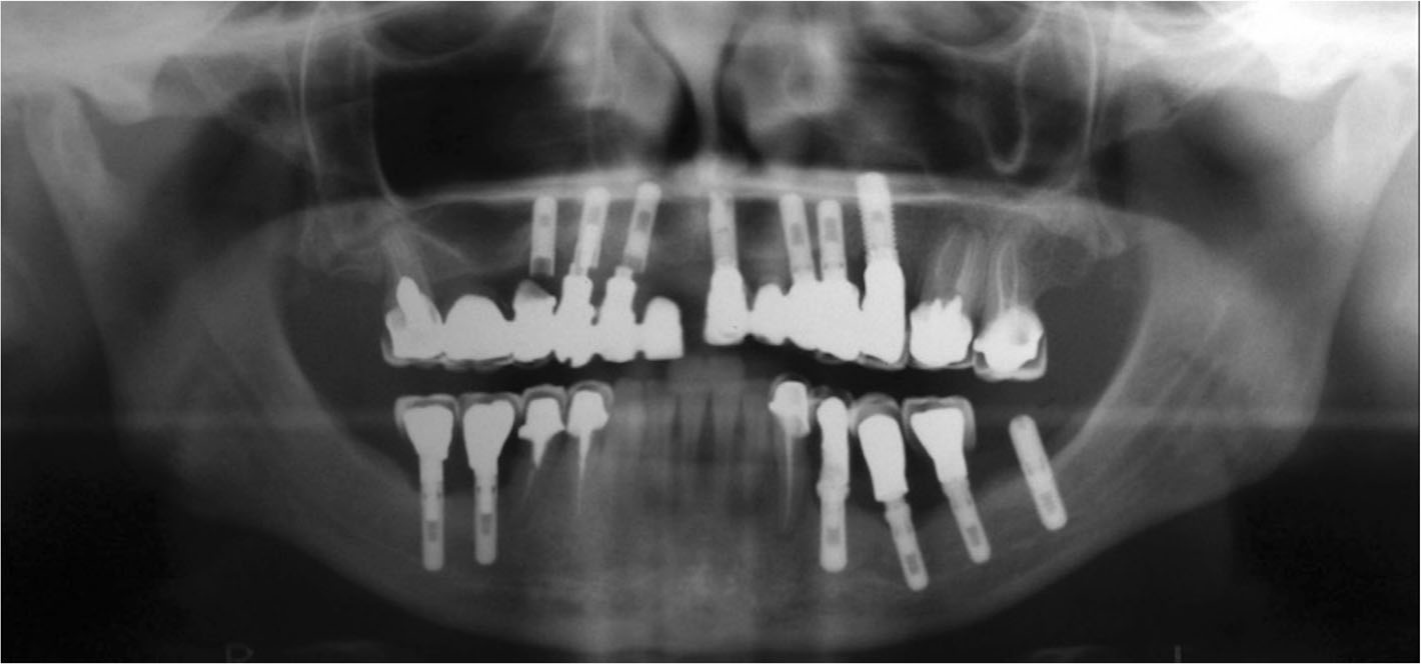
Radiograph of the fractured implants united to a tooth after 45 months in function
